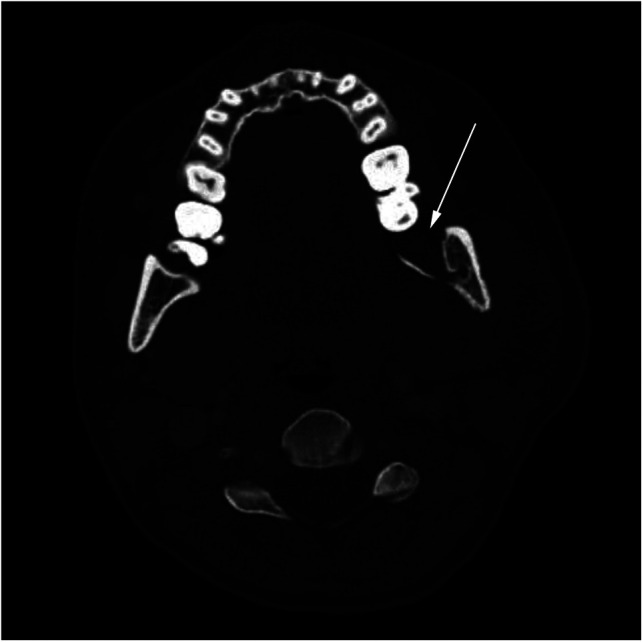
Preoperative axial CT scan of the neck at the C2 level showing osteomyelitis in region 38 of the mandibular ramus. The affected side shows loss of cortical margin (arrow) and soft tissue swelling.
